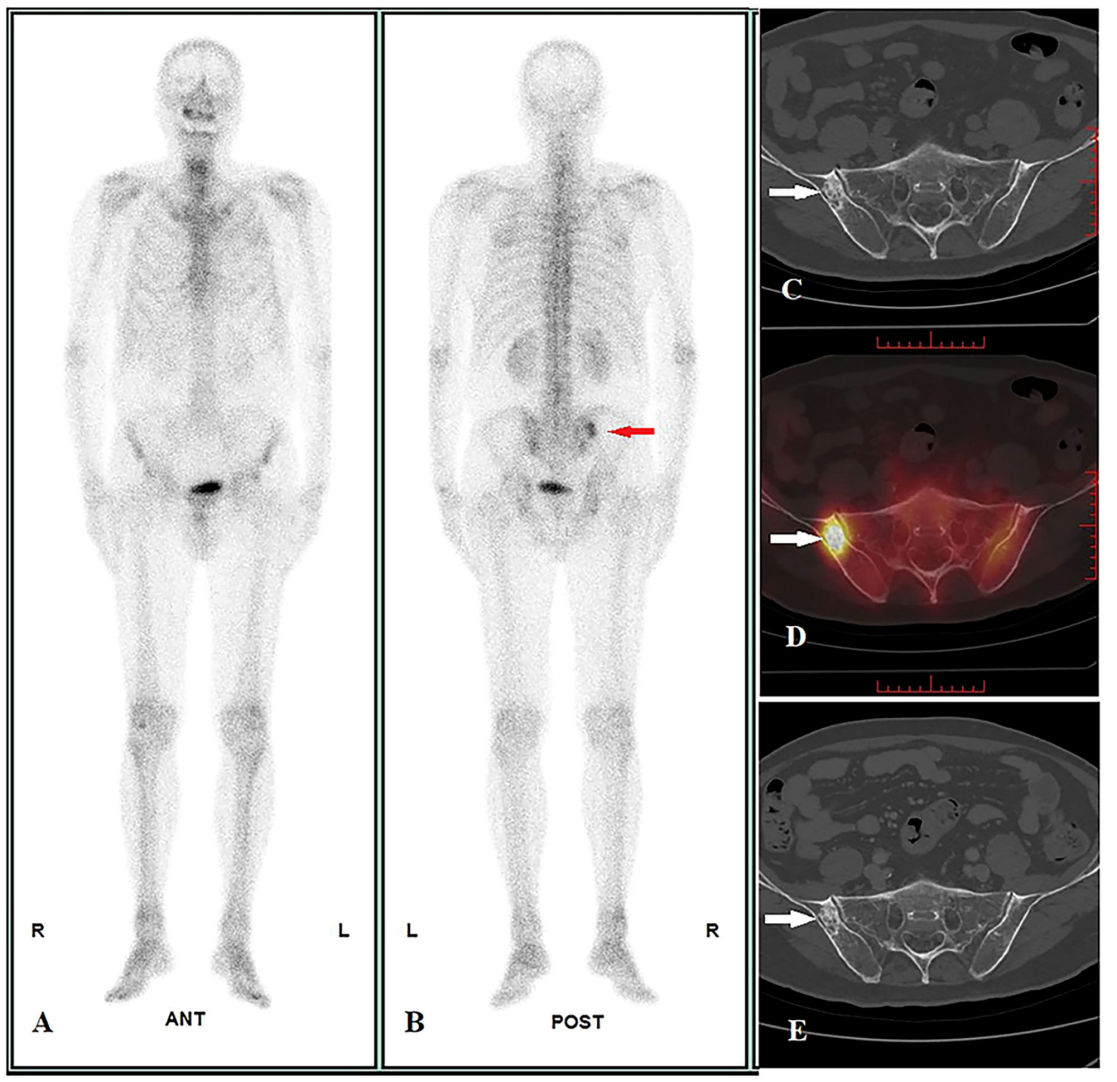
Image display of true negative cases. A 70-year-old male pulmonary adenosquamous carcinoma patient performed WBS and quantitative SPECT/CT imaging before treatment on 07/01/2022. WBS showed abnormal radioactivity concentration in the right ilium at the posterior (A: anterior, B: posterior, red arrow), and the SUVmax of lesion was 15.60 according to quantitative SPECT/CT (D, white arrow); the CT images showed osteoblastic change, but the diagnosis cannot be confirmed (size = 2.6 cm * 1.6 cm, average CT value = 650 HU) (C, white arrow). After regional radiotherapy to the chest, systemic chemotherapy, and immunotherapy, a posttreatment CT scan showed new metastasis with the liver and L1 on 14/10/2022 (not shown in the picture). However, at follow-up until 18/03/2023, the size and average CT value of the lesion did not show any significant change on CT images (size = 2.6 cm * 1.6 cm, average CT value = 648HU) (E, white arrow), suggesting a benign bone lesion.
